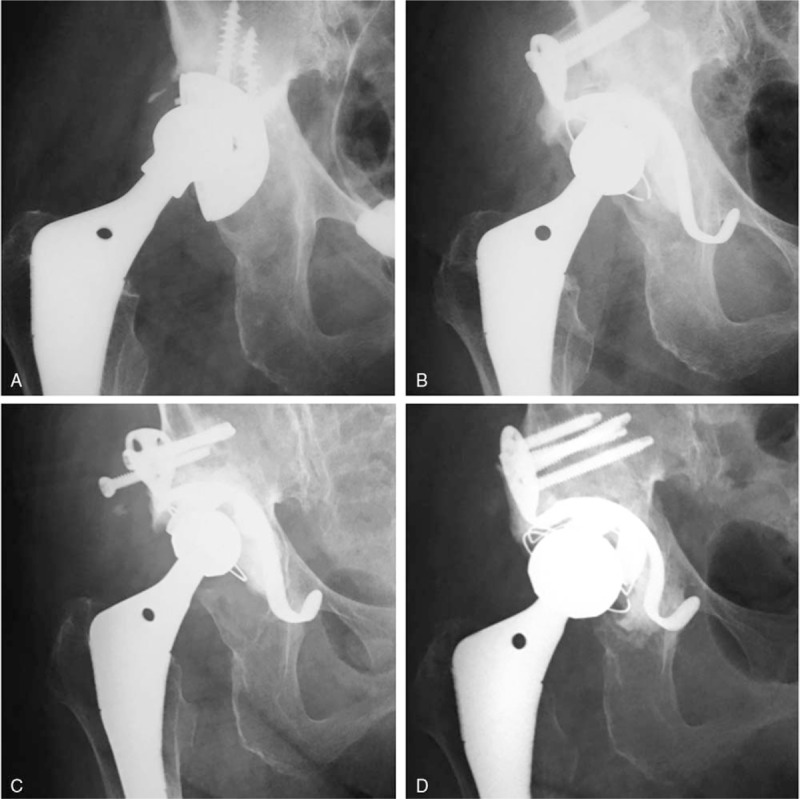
X-ray radiograph of the hip with 77-year-old woman. A. at initial visit. B. after first revision surgery with HH-type KT and a morselized bone graft. C. nine years after revision surgery. D. after re-revision surgery with OC-type KT and a structural bone graft. HH = high hip center-type, KT = Kerbull-type acetabular support rings, OC = original cup center-type.
